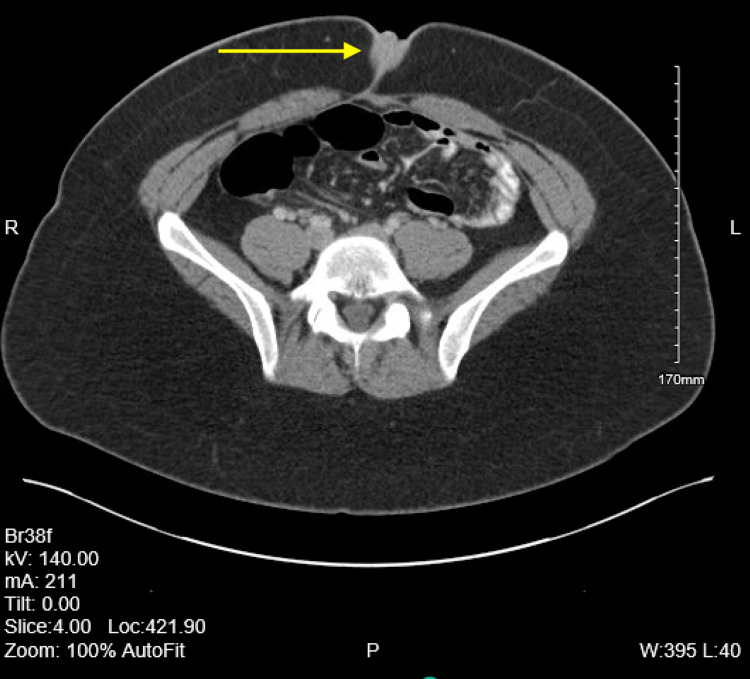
Computerized tomography (CT) scan 3
